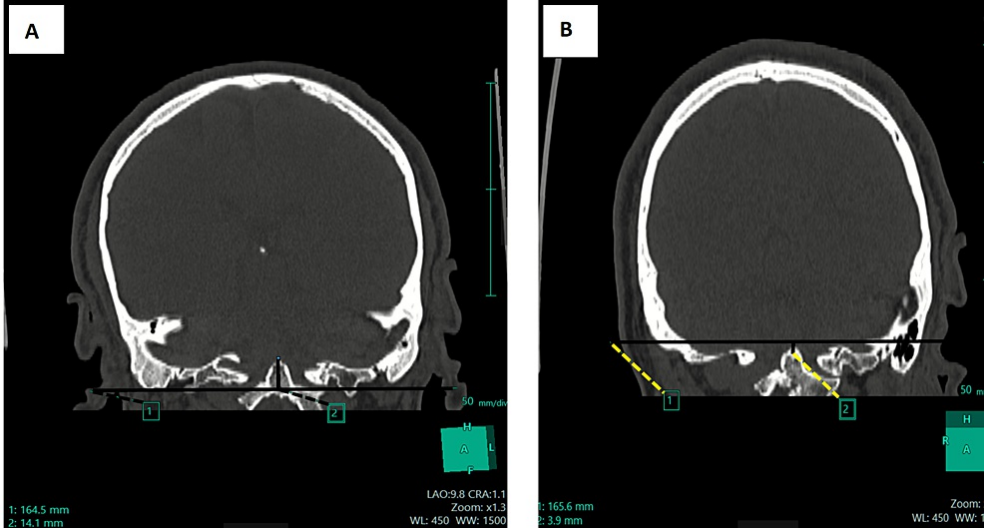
Coronal CT images show that the tip of the odontoid process is 14.1 mm above the bimastoid line (A). The tip of the odontoid process is at a distance of 3.9 mm from the digastric line (B).
